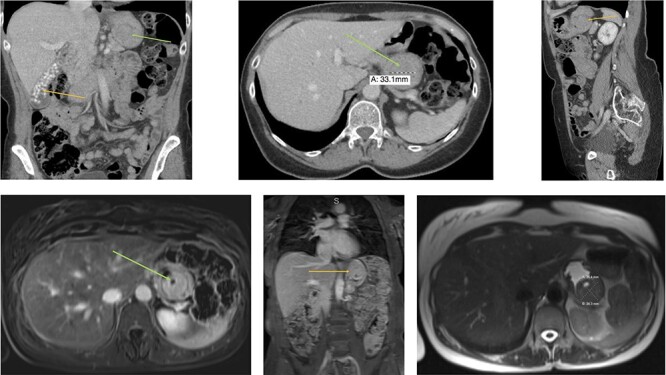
Radiology (a) CT scan coronal view showing the gallbladder filled with radiolucent stones (orange arrow) and the GIST in the stomach (green arrow). (b) CT scan axial view showing the gastric GIST in the submucosal tissue (green arrow). (c) CT scan sagittal view showing the gastric GIST on the posterior wall of the stomach (orange arrow). (d) MRI axial view showing the tumour with a pneumatocele (green arrow). (e) MRI coronal view showing the tumour on the posterior wall of the stomach (orange arrow) with the visible pneumatocele. (f) T2 weighted MRI axial view showing the GIST dimensions.
